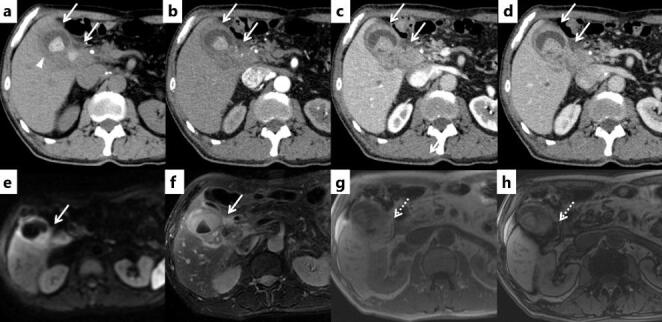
Xanthogranulomatous cholecystitis (XGC). a–d Dynamic contrast-enhanced CT: a Plain CT, b arterial phase, c portal phase, d equilibrium phase, e–h MRI: e DWI, f fat-suppressed T2WI, g–h chemical shift imaging: g in phase, h opposed phase. A man in his 70s with type 2 DM underwent CT evaluation for elevated hepatobiliary enzyme levels and chronic abdominal pain. Contrast-enhanced CT (a–d) showing gallstones (a; arrowhead) and diffuse thickening of the gallbladder wall (a–d; arrow). MRI shows diffusion restriction in the wall of the gallbladder on DWI (e; arrow). Chemical shift imaging showing low fat intensity (g–h; dotted arrow). A cholecystectomy was performed, and the patient was diagnosed with XGC. Abbreviations: XGC, xanthogranulomatous cholecystitis; CT, computed tomography; MRI, magnetic resonance imaging; DWI, diffusion-weighted imaging; T2WI, T2-weighted imaging
